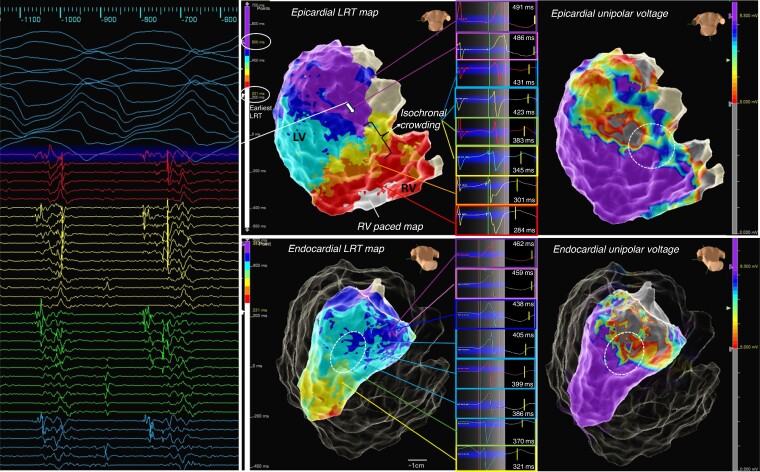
Example of iREM: a patient with left-sided ACM, inferolateral mid- and subepicardial scar in MRI, fast VT, and episodes of ventricular ectopy-triggered VF. High-density repolarization mapping was performed as described, with LRT map shown and total repolarization time displayed over eight isochrones (here 221 to 500 ms). A zone of isochronal crowding was identified at the inferior base at the border zone of the unipolar low-voltage area (white circle) allowing to quickly identify an area of abnormally steep repolarization gradients leading towards a zone of substantially prolonged repolarization corresponding to the area of scar as indicated in the voltage map. Bottom The corresponding LV endocardial LRT map equally displays a zone of isochronal crowding at the border zone in the mid-inferolateral segment albeit much more discrete. A fast VT (tachycardia cycle length 250 ms) was induced with loss of cardiac output, the HD grid overlaying the area of late repolarization adjacent to the iREM area recorded mid/end-diastolic potentials, with ATP the VT degenerated to VF and required DCCV. Activation sequence of the HD grid suggested conduction from area of late activation towards iREM.
